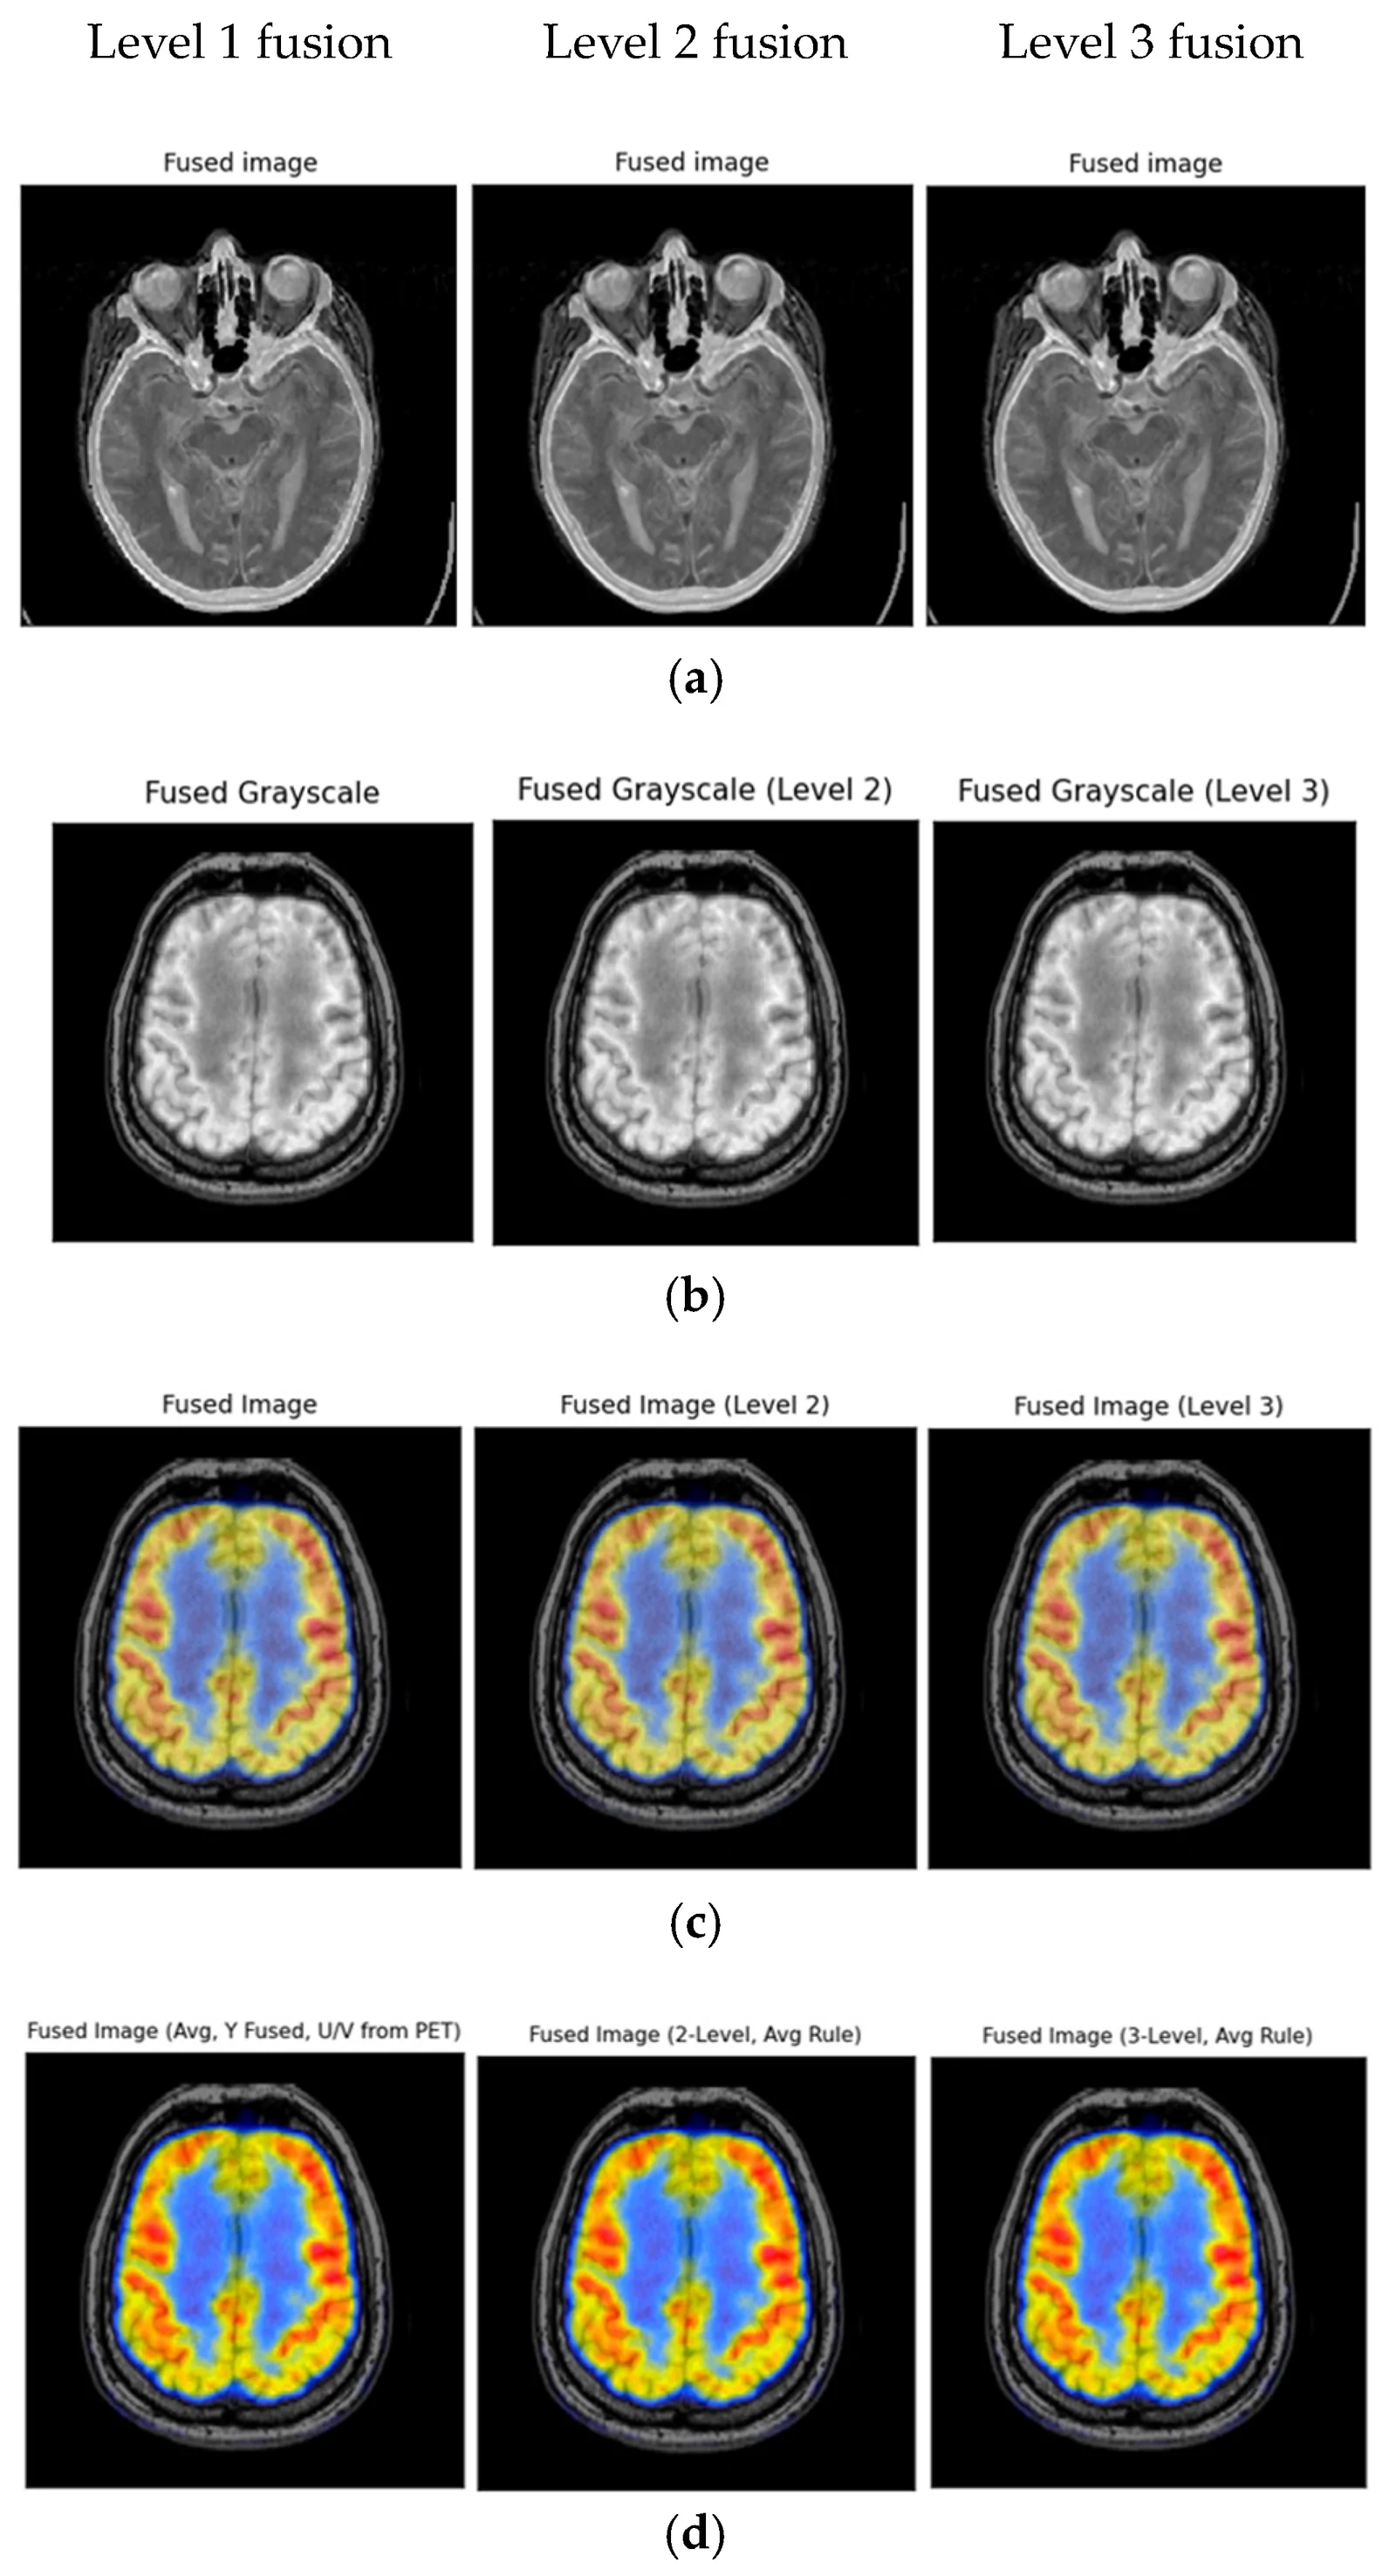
Comparison of one-, two-, and three-level fusion results, using the average fusion rule with the ‘bior4.4’ wavelet: (a) CT and MRI; (b) PET grayscale and MRI; (c) PET without conversion and MRI; (d) PET YUV conversion and MRI.
